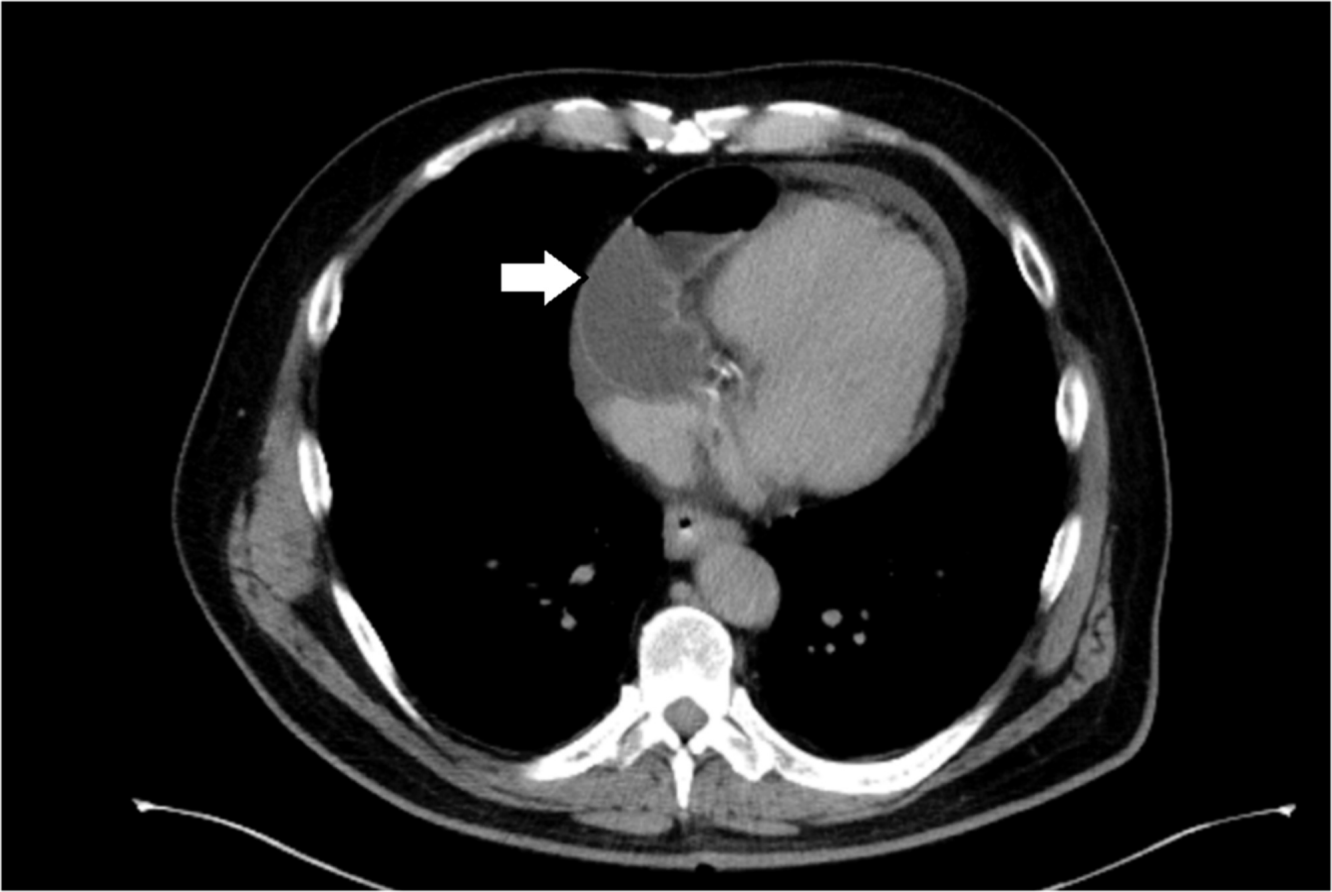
Computed tomography demonstrating a small bowel loop next to the heart in the pericardial sac
